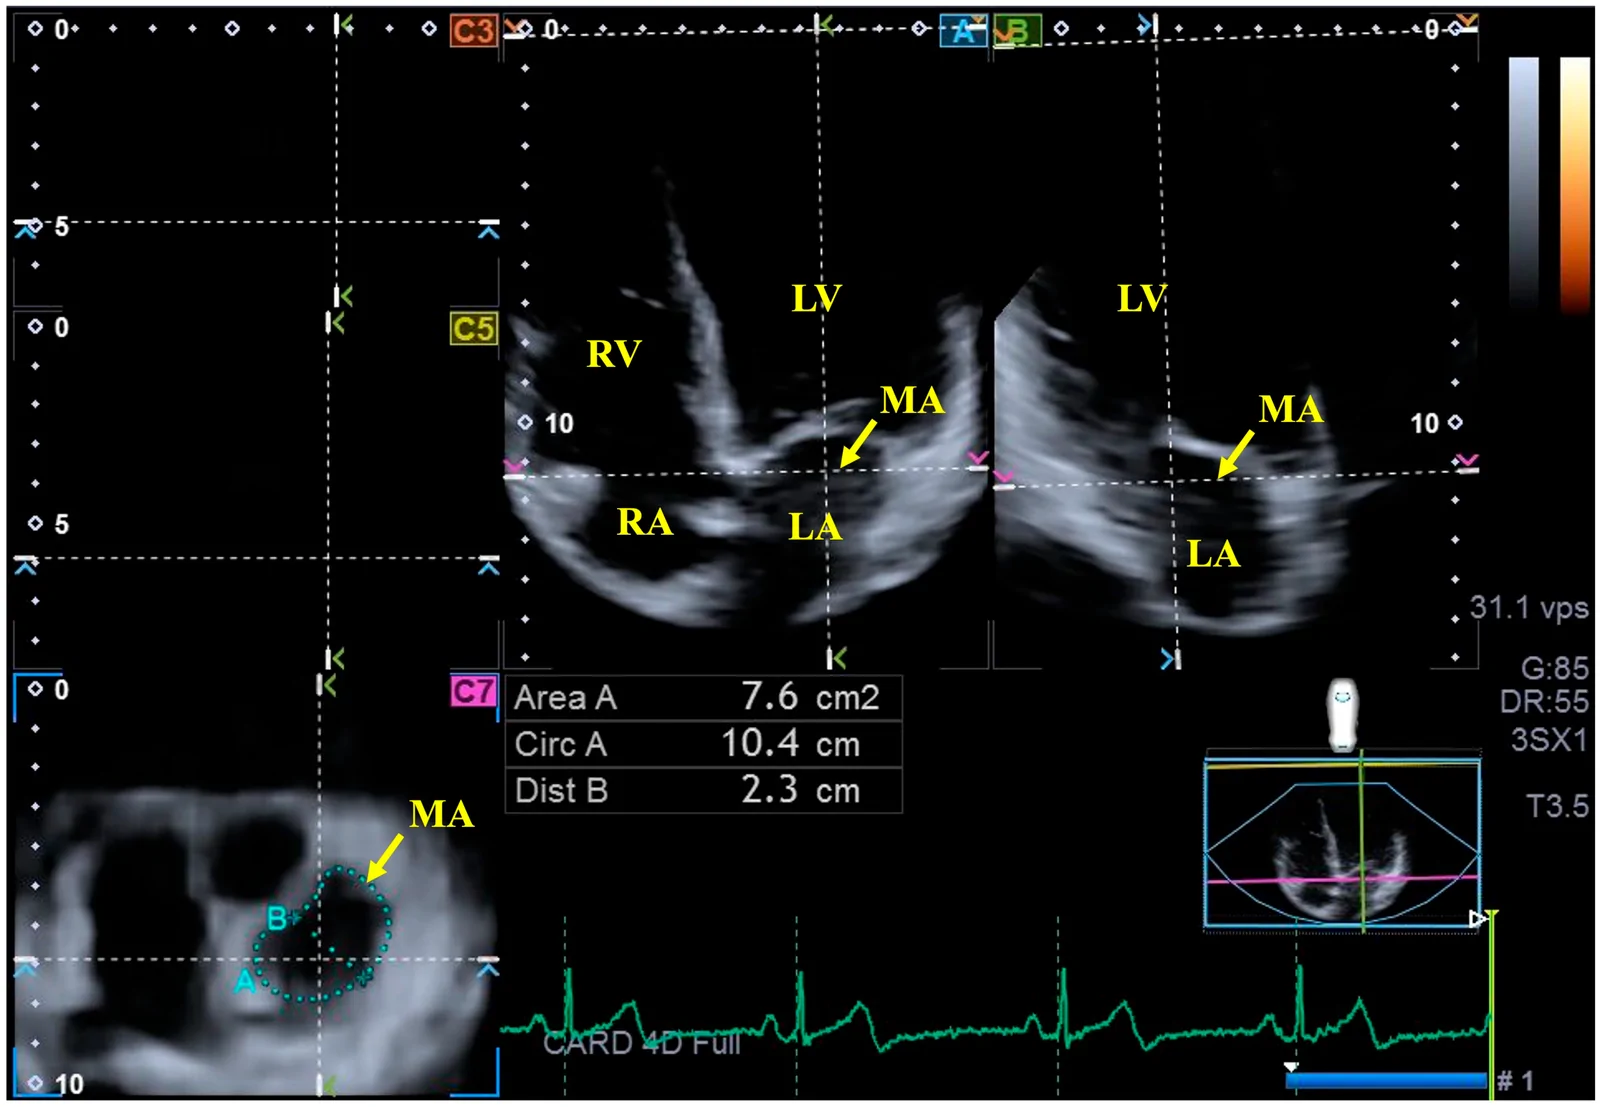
A three-dimensional speckle-tracking echocardiography-derived full-volume dataset displaying the mitral annulus in a representative case: (A) apical four-chamber view, (B) apical two-chamber view, and (C7) a short-axis cross-sectional view at the level of the mitral annulus, optimized using the apical four- and two-chamber long-axis views. The yellow arrow delineates the mitral annular plane across both the long-axis (A, B) and short-axis (C7) projections. Abbreviations: Area = mitral annular area, Circ = mitral annular perimeter, Dist = mitral annular diameter, LA = left atrium, LV = left ventricle, MA = mitral annulus, RA = right atrium, RV = right ventricle.
